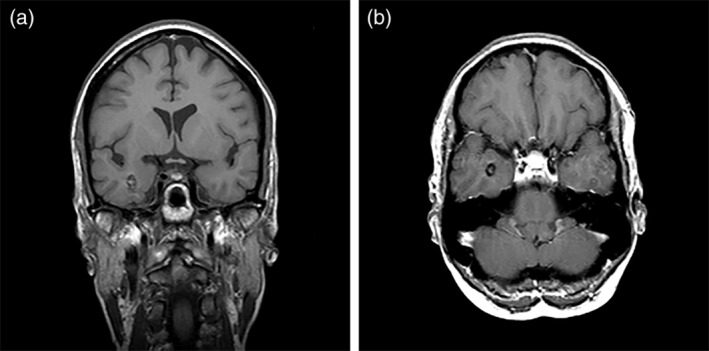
MRI scans taken 1 year before surgery (a: coronal view, b: axial view). Note the location of the cavernous hemangioma (measuring 9 mm in diameter) in the right medial temporal lobe directly lateral to the head of the hippocampus. There was no sign of hippocampal sclerosis. The right side of the brain corresponds with the left side
